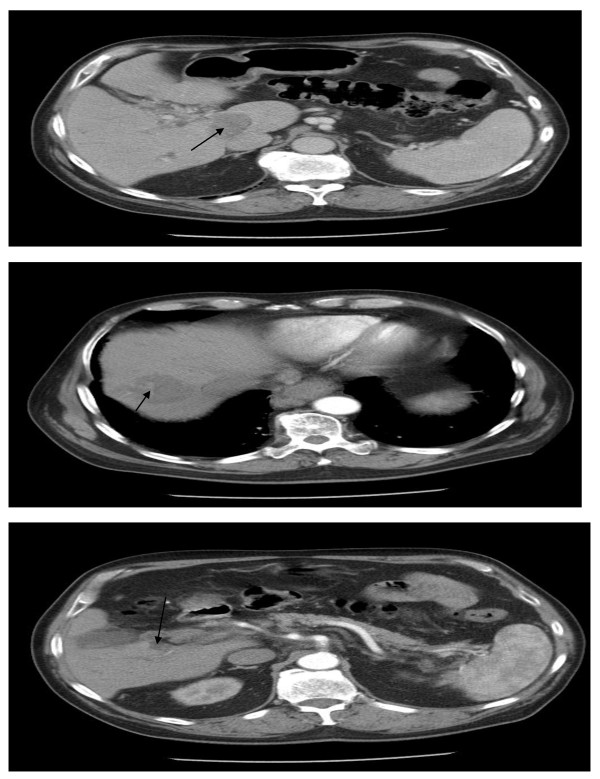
(A-C) CT scans (January 2008): HCC lesions in caudate lobe, segment 7 and segment 5 of liver, respectively.
